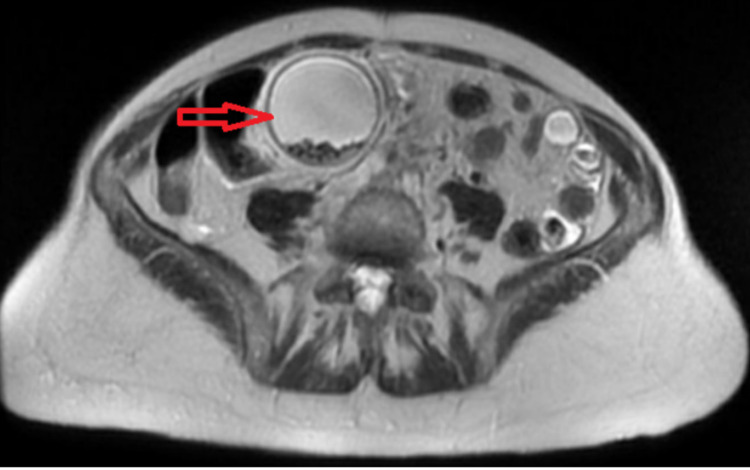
MRI of the abdomen showing the gallbladder hydrops (arrow) MRI: magnetic resonance imaging
